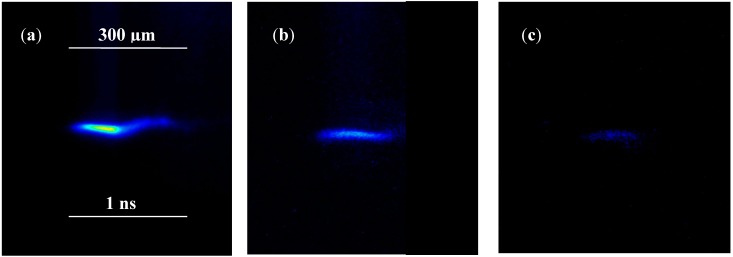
X-ray CCD streak camera images of CD_2_ plasma obtained irradiating in the same experimental conditions a high density deuterated polyethylene foil 50 µm in thickness in TNSA regime (a), a low density foam foil, 3 mm in thickness, (b) and a high density 3 mm deuterated foil irradiated in BPA regime (c).
